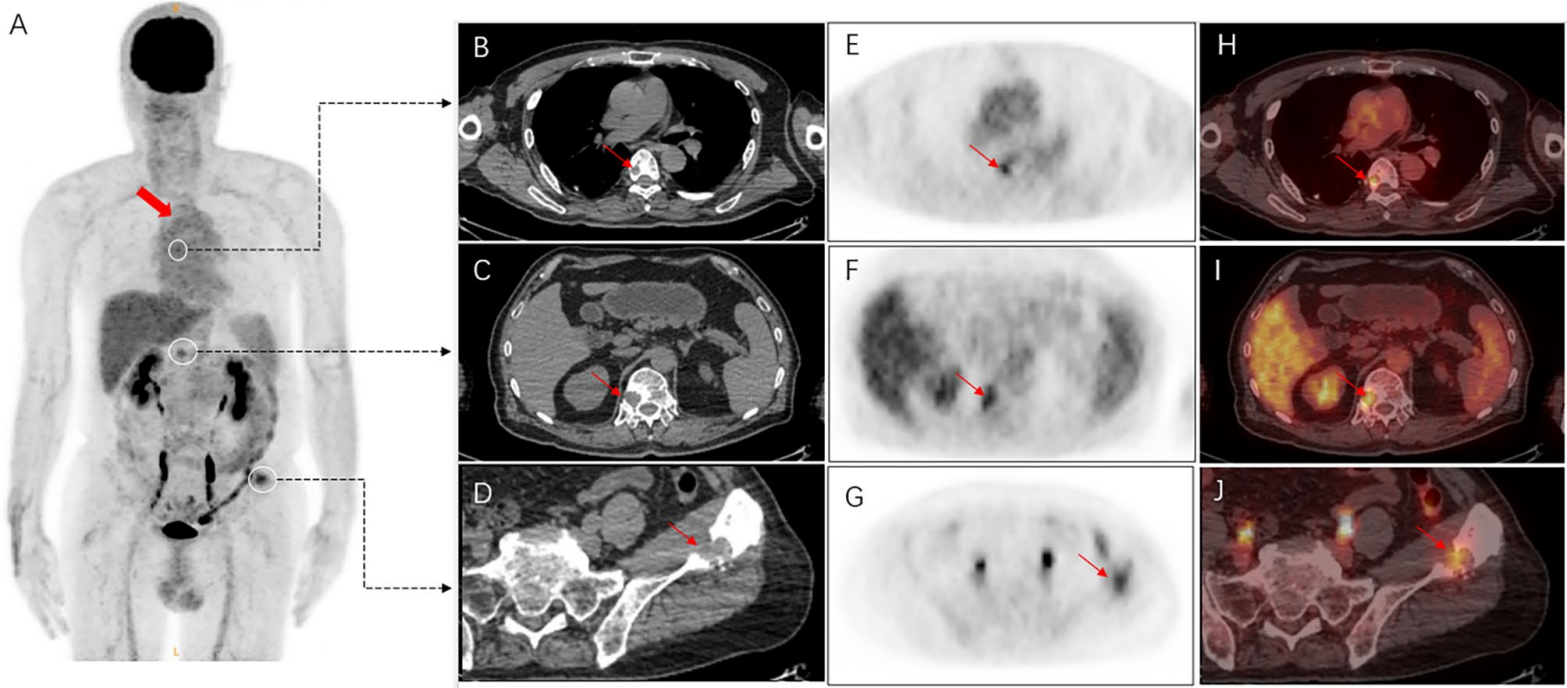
^18^ F-FDG PET/CT identified multiple lesions located in the T6, T12 vertebrae and the left ilium (A, white circle), all of which showed osteolytic bone destruction on CT (B-D). The maximum density projection (MIP) PET (A), corresponding PET (E-G), and fusion images (H-J) showed increased FDG uptake in these lesions (arrows) with a SUVmax of 4.9. No abnormal FDG uptake was shown on the manubrium sterni (A, red arrow)
